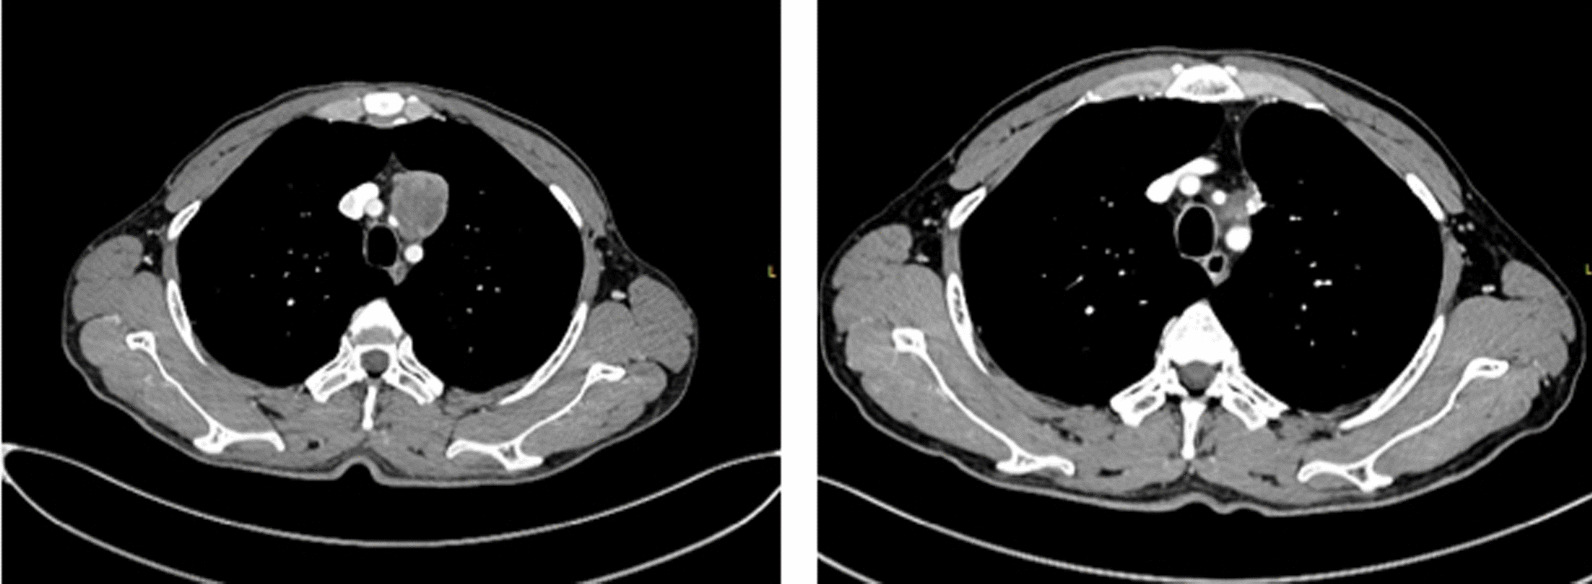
Initial enhanced CT scan of the upper mediastinum demonstrated a large anterior mediastinal mass attached to the adjacent vessels with heterogeneous enhancement (left). Post-treatment enhanced CT scan of the upper mediastinum showed residual soft tissue of the anterior mediastinum consistent with postoperative changes (right)
